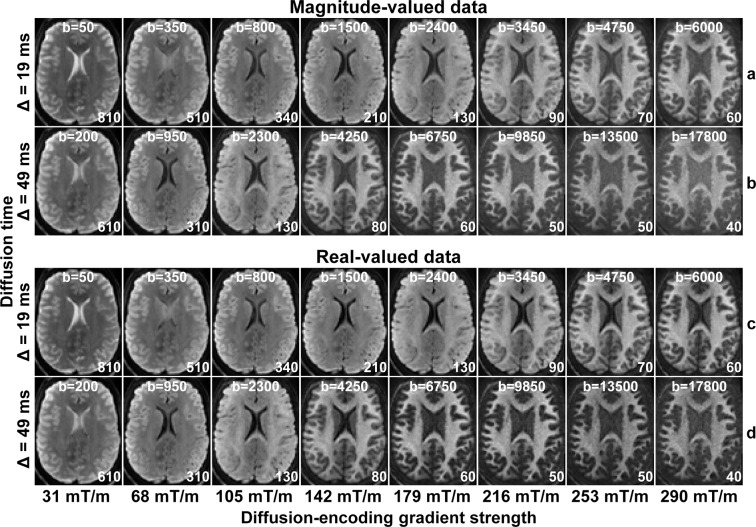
Spherical mean diffusion-weighted images. Spherical mean diffusion-weighted images for magnitude-valued (rows a, b) and real-valued (rows c, d) diffusion MRI data for each diffusion time (Δ) and diffusion-encoding gradient strength of a representative healthy participant. The images are windowed individually for improved visualization, between 0 and a higher image intensity listed at the bottom right of each sub-figure.
